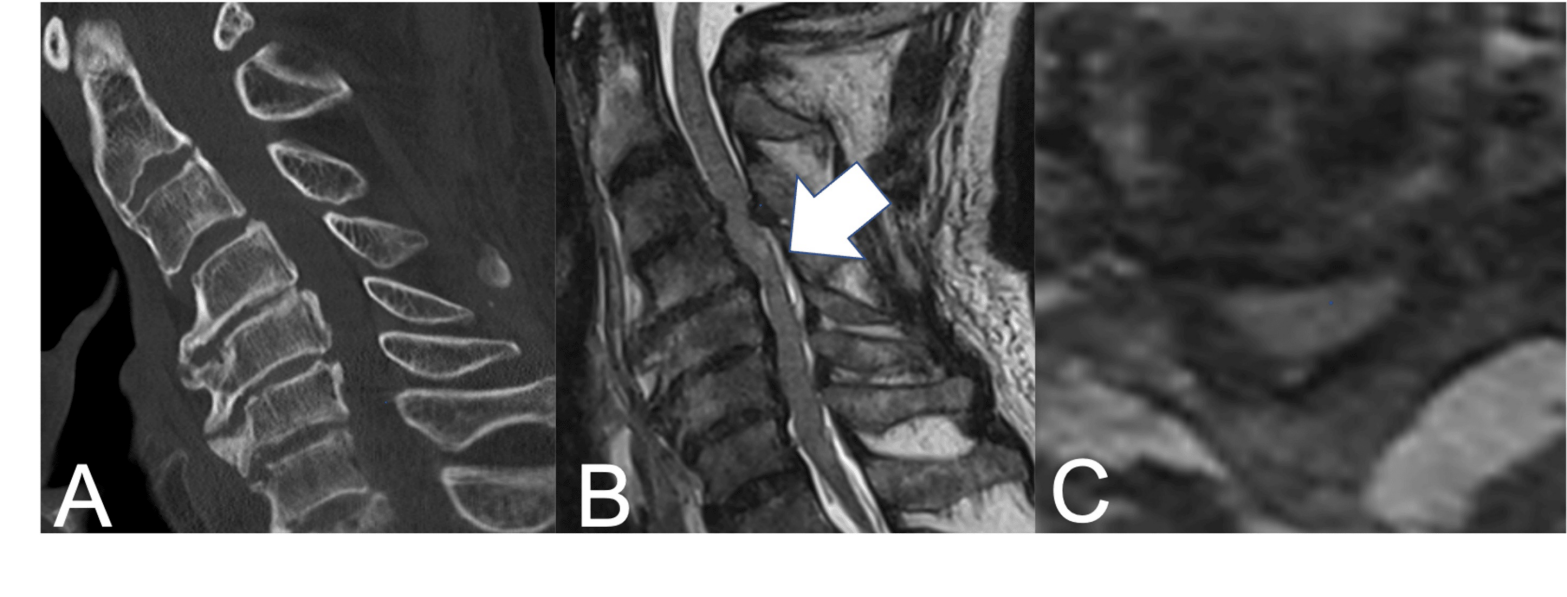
(A) Sagittal CT scan at admission show no bone injury; (B) Sagittal T2-weighted MRI on admission indicate mild intramedullary signal change at the C3/4 level (white arrow); (C) Axial T2-weighted MRI at admission show severe cervical spinal canal stenosis.
